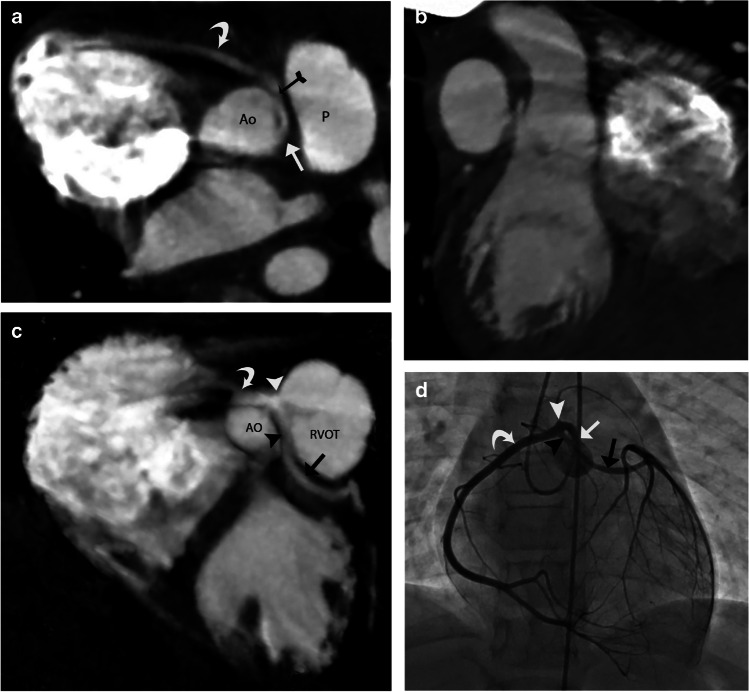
A 9-year-old male patient who presented with cardiac arrest was noted to have a single coronary artery arising from the left sinus of Valsalva with its interarterial intramural course and additional transseptal course of the left main coronary artery (LMCA). a Coronary CT angiography (CTA), oblique axial image. A single coronary artery arises from the left sinus of Valsalva (white arrow), followed by an interarterial course between the aorta (Ao) and pulmonary artery (P) with an intramural segment. It has a hyperacute angle of origin (white arrow) and a course along the aorta. The black-tailed arrow points to the exit point of the intramural segment. The curved white arrow points to the normal course of the right coronary artery (RCA), the branch of the anomalous single coronary artery. b Coronary CTA, oblique coronal image. An elliptical luminal shape (white arrow) of the interarterial intramural single coronary artery is noted, consistent with compression. c Coronary CTA, oblique coronal image. The white arrowhead points at the bifurcation of the single coronary artery after it exits out of the intramural segment at the anterior right sinus into the RCA (curved white arrow) and LMCA (black arrowhead). The LMCA extends between the aorta (Ao) and right ventricular outflow tract (RVOT), followed by the transconal course (black arrow) as it dips into the conal septal myocardium. d Catheter angiography frontal view with selective catheter injection of the single coronary artery at the left sinus of Valsalva. The single coronary artery (white arrow), after originating from the left sinus of Valsalva, extends towards the right side, indicating its interarterial course. The white arrowhead points to the bifurcation of the single coronary artery into RCA (curved white arrow) and LMCA (black arrowhead). The LMCA then dips down like a hammock at the expected level of the RVOT, consistent with its transseptal course (black arrow). The LMCA then branches out at the lateral margin of the heart
